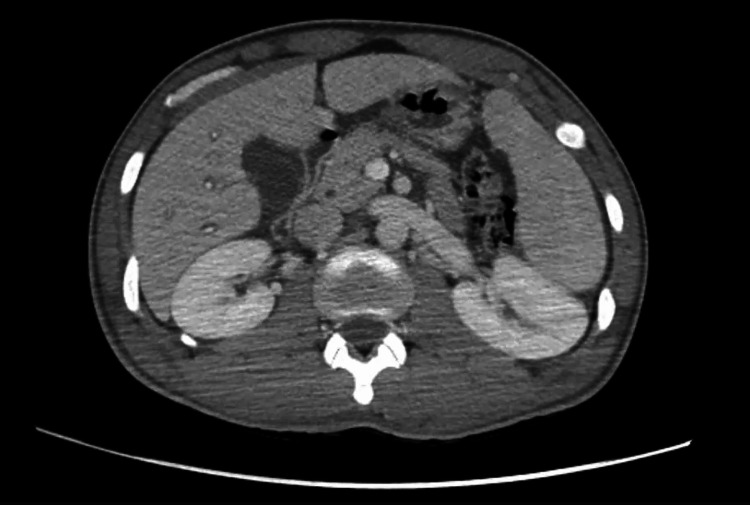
CT scan of the abdomen and pelvis This image depicts generalized edema, slight ascites, periportal edema in the liver, pericholecystic edema, hepatomegaly, and splenomegaly.
